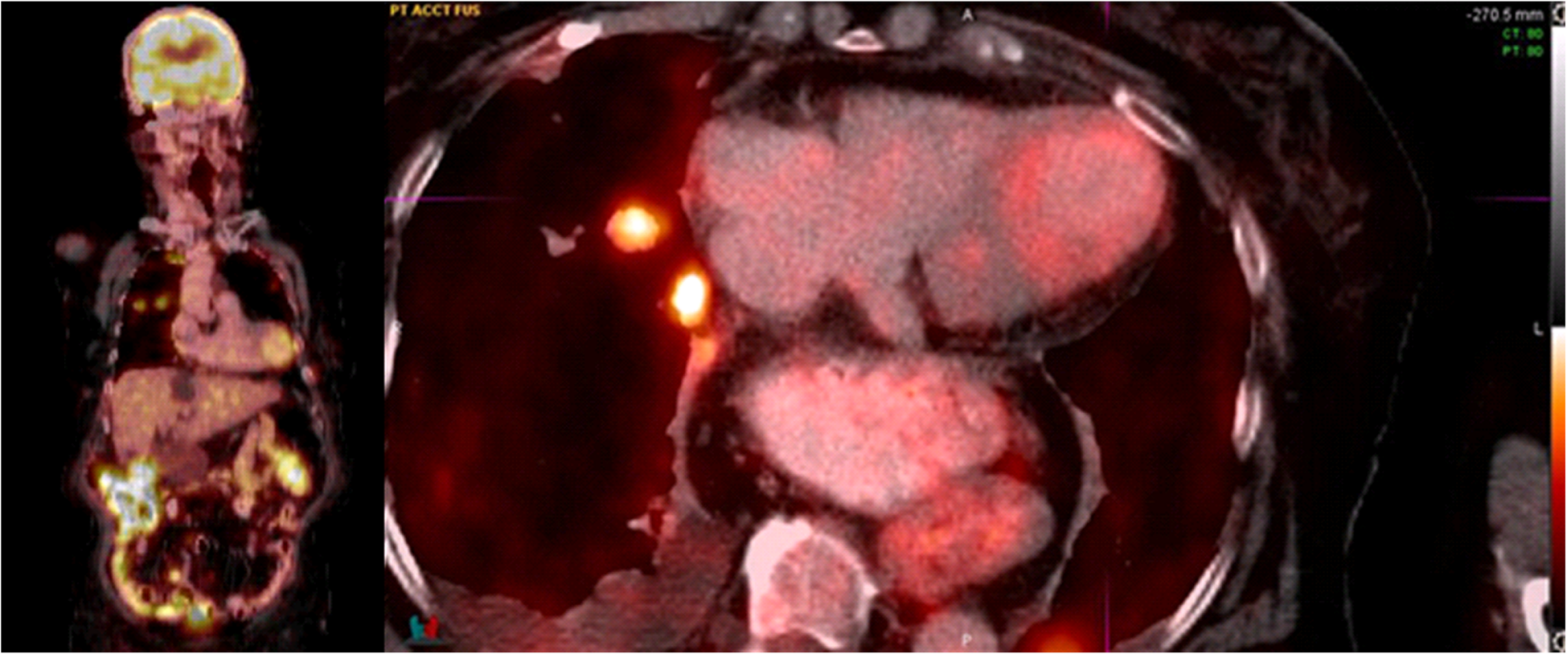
Coronal (left) and axial (right) PET/CT showing a focus of mildly increased FDG uptake corresponding to ballooning of the left ventricular apex. Additional findings at the axial level through the chest include FDG-avid metastatic lung nodules in the right upper lobe, small right pleural effusion and a large hiatal hernia.
